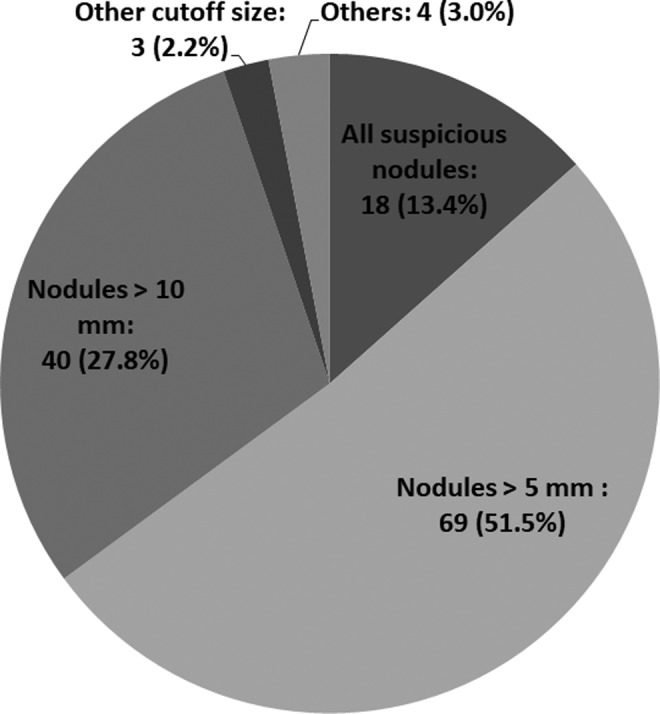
Indication of fine-needle aspiration cytology for thyroid nodules with suspicious ultrasound features.
